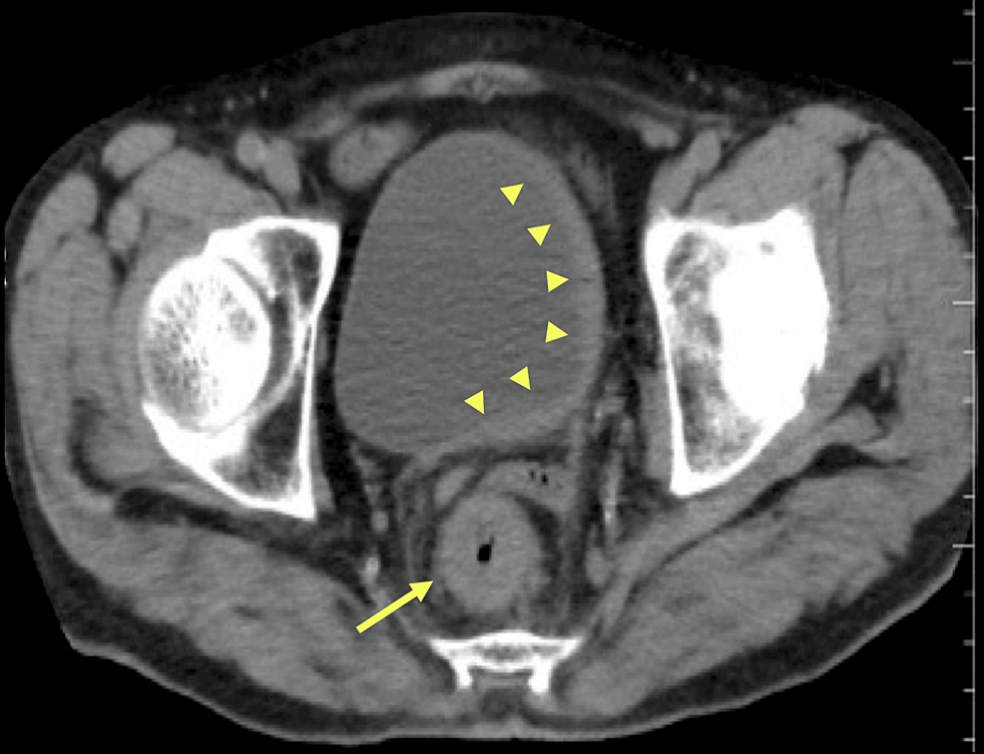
Abdominal contrast-enhanced CT findings. Asymmetric thickening of the bladder wall and rectal wall was observed on abdominal contrast-enhanced CT.
